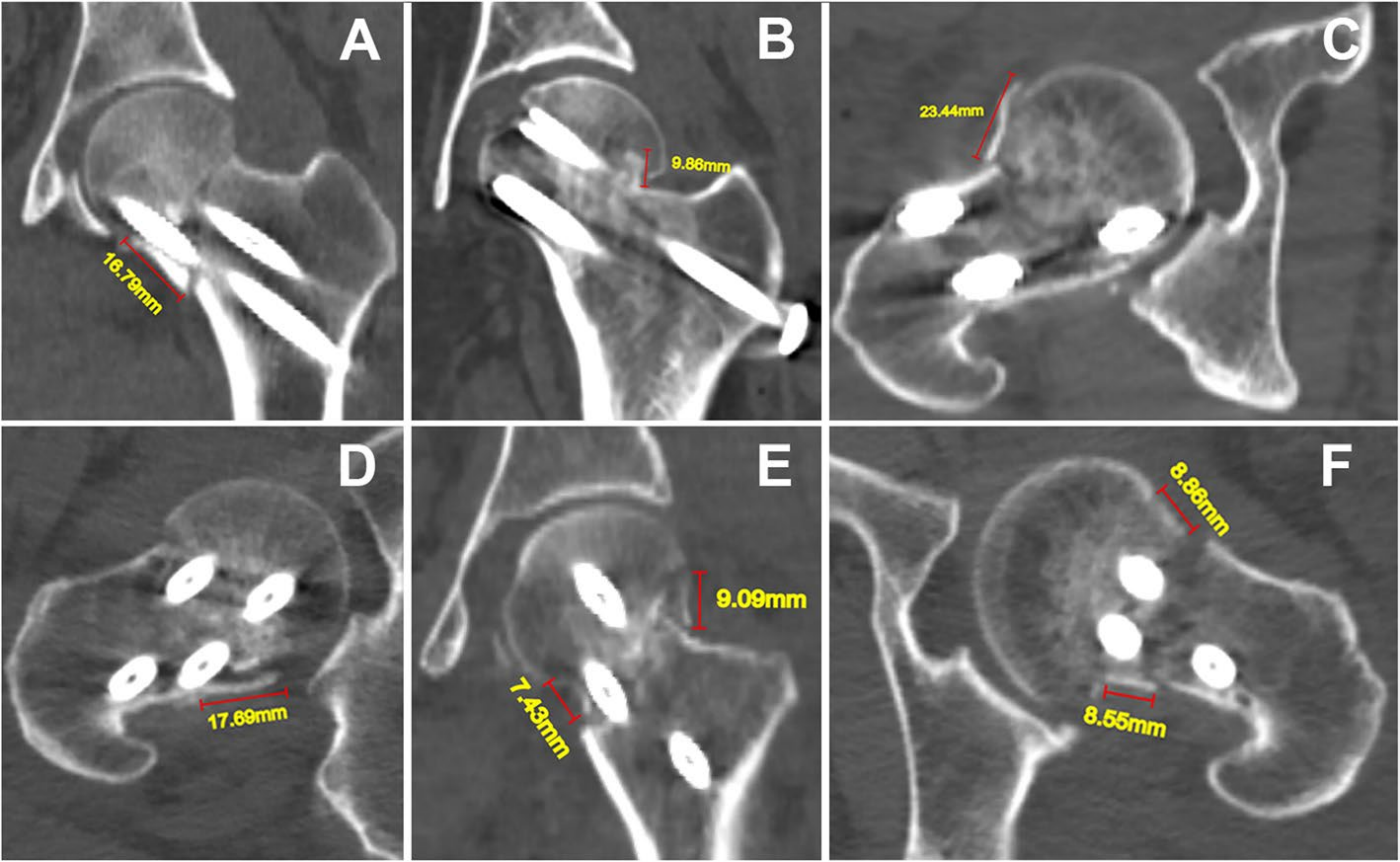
Radiographic measurements of cortical comminution in coronal and axial planes. A and B. Isolated comminutions of the inferior and superior cortex. C and D. Unilateral comminutions of the anterior and posterior cortex. E. Combined comminution of both inferior and superior cortices. F. Bilateral comminution of both anterior and posterior cortices
